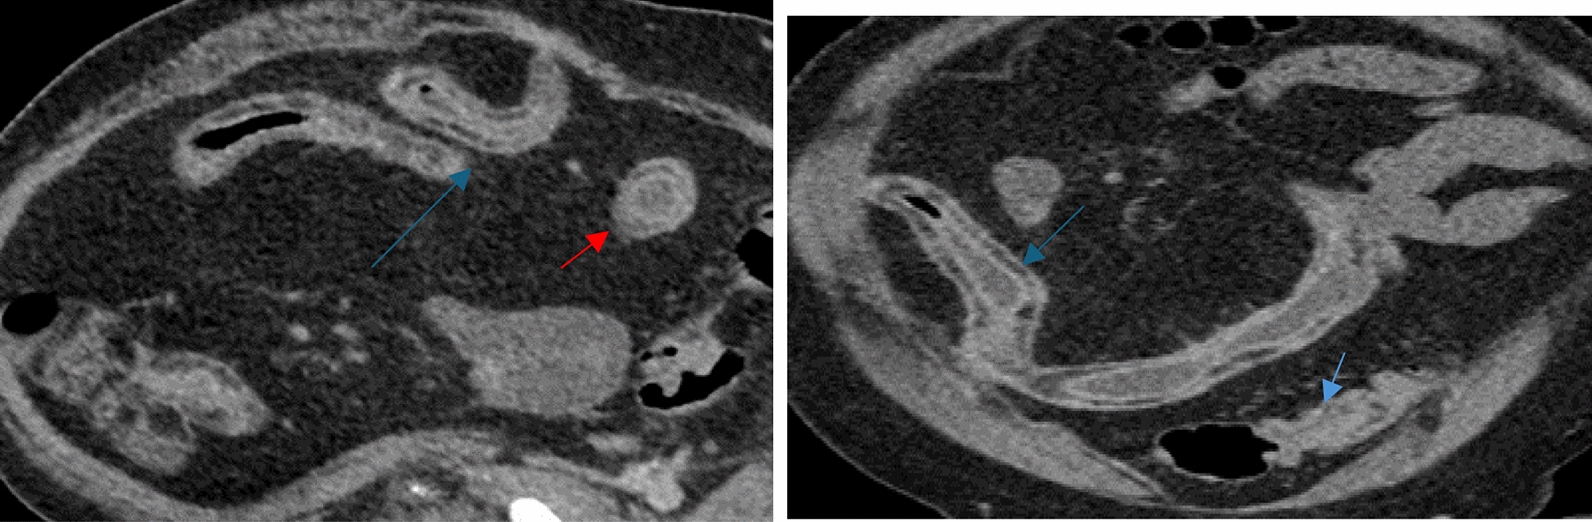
Axial and coronal CT images of the distal ileum showing extensive submucosal fat deposition (red arrrow) corresponding with sequela of chronic and severe inflammation in a 62-year-old patient with Crohn’s disease. Also, prominent regional fibrofatty proliferation separating the loops of bowel, “creeping fat” sign (blue arrow), typical of Crohn’s disease
